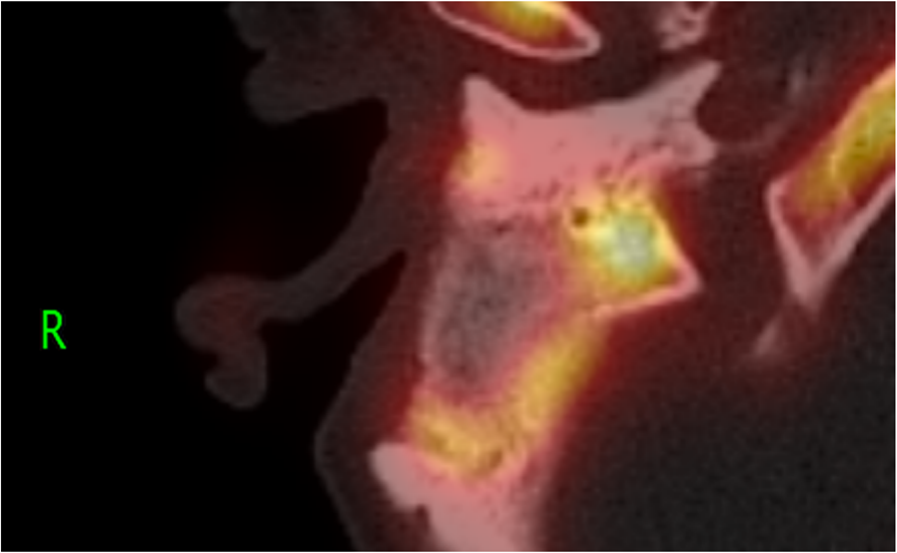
18F-NaF PET/CT showing increased uptake of 18F-NaF at the edges of the obliterated cavity. It was considered as osteoblast activity induced by bioactive glass S53P4 indicating formation of new bone
